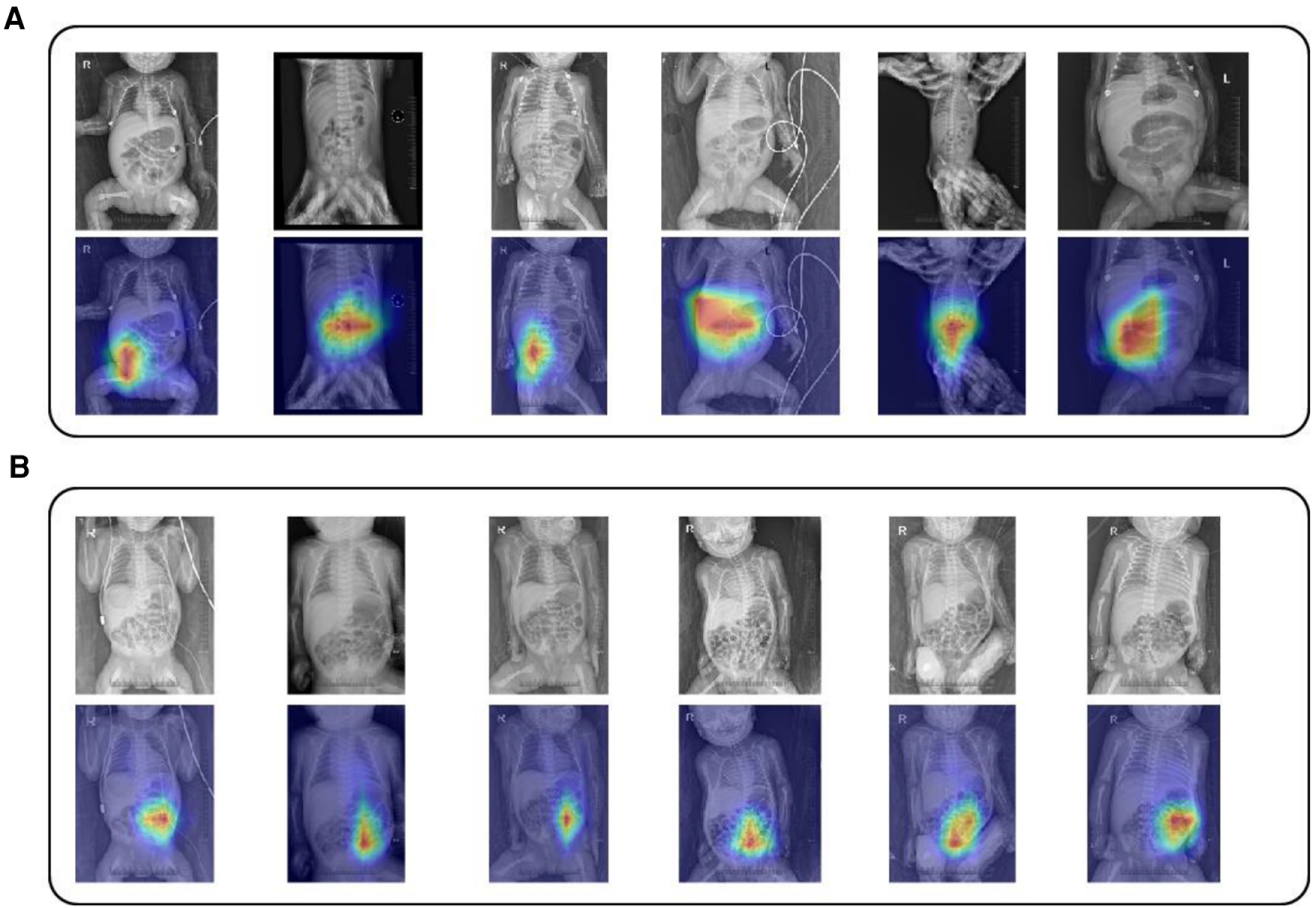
ResNet34-Clinical grad-CAM images (A) Overlaid images of abdominal x-rays and GradCAM for NEC patients. (B) Display of overlaid images of abdominal x-rays and GradCAM for non-NEC newborns. The thicker red area indicates the image portions that ResNet34-Clinical focuses on during the classification of NEC patients and healthy non-NEC newborns.
